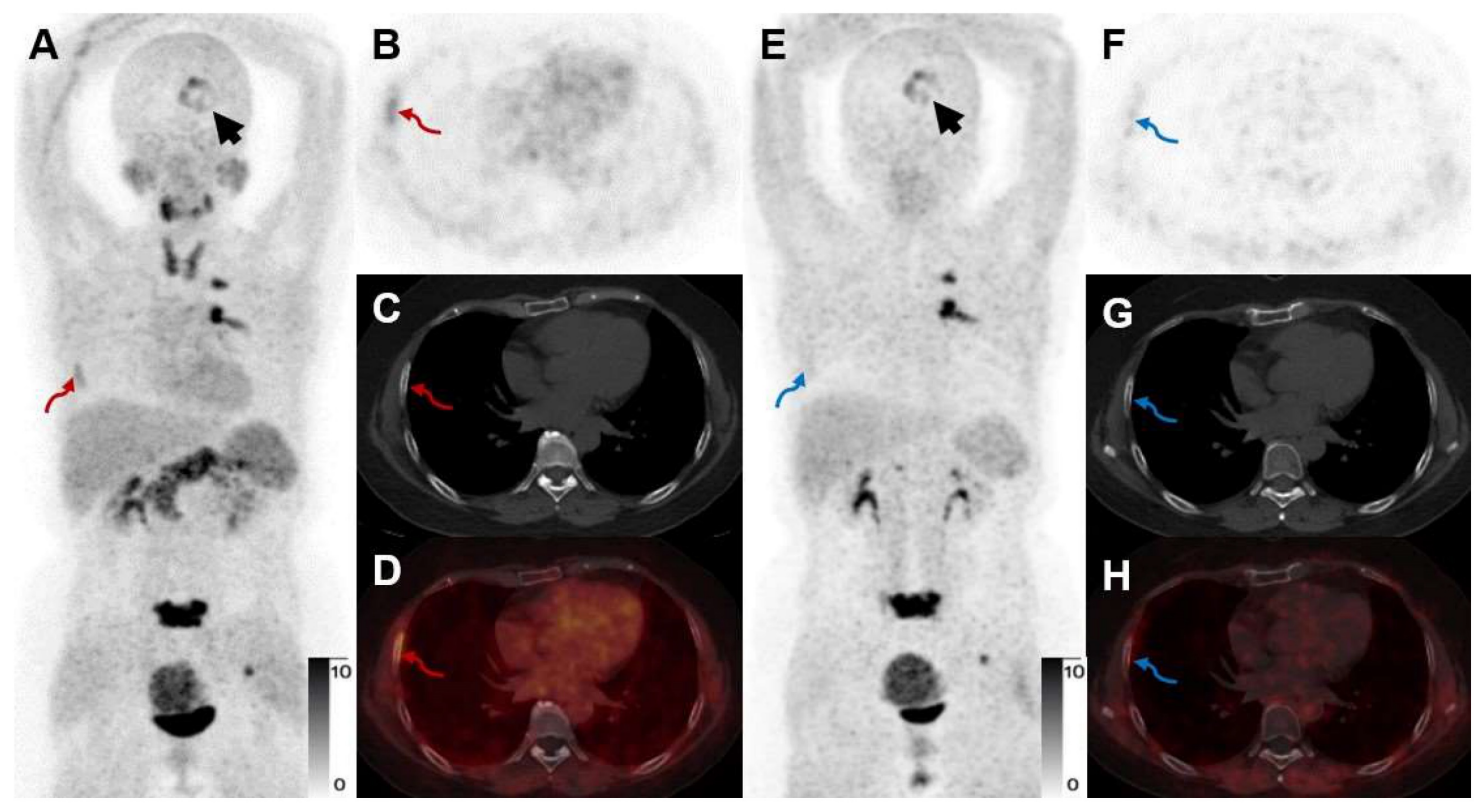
A 52-year-old woman with IAC (Participant No. 44) underwent two PET scans. [^68^Ga]Ga-FAPI-RGD showed higher uptake in brain metastasis than [^68^Ga]Ga-FAPI (SUVmax, 7.7 *vs.* 5.6, black arrows). One more rib lesion with high uptake was found (A-D, red arrows, SUVmax 4.5) by [^68^Ga]Ga-FAPI-RGD PET/CT while not detected by [^68^Ga]Ga-FAPI PET/CT (E-H, blue arrows), which had been confirmed as a metastatic lesion on previous whole-body bone scan.
